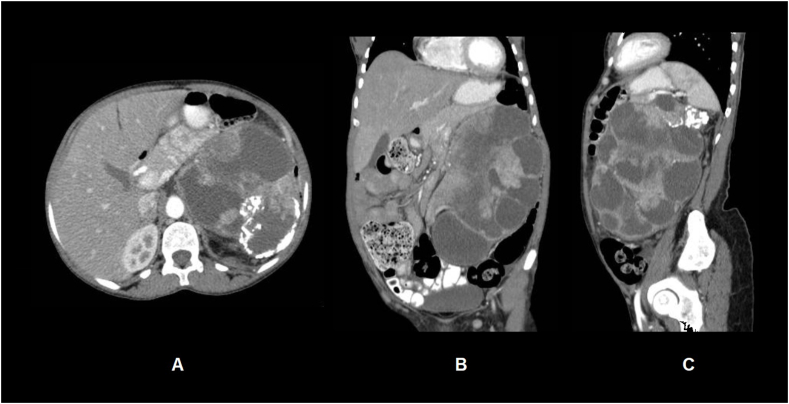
Axial (A), coronal (B) and sagittal (C) views of the computed tomography of the abdomen show a large heterogenous mass of the left kidney with solid, cystic, and calcific components.
